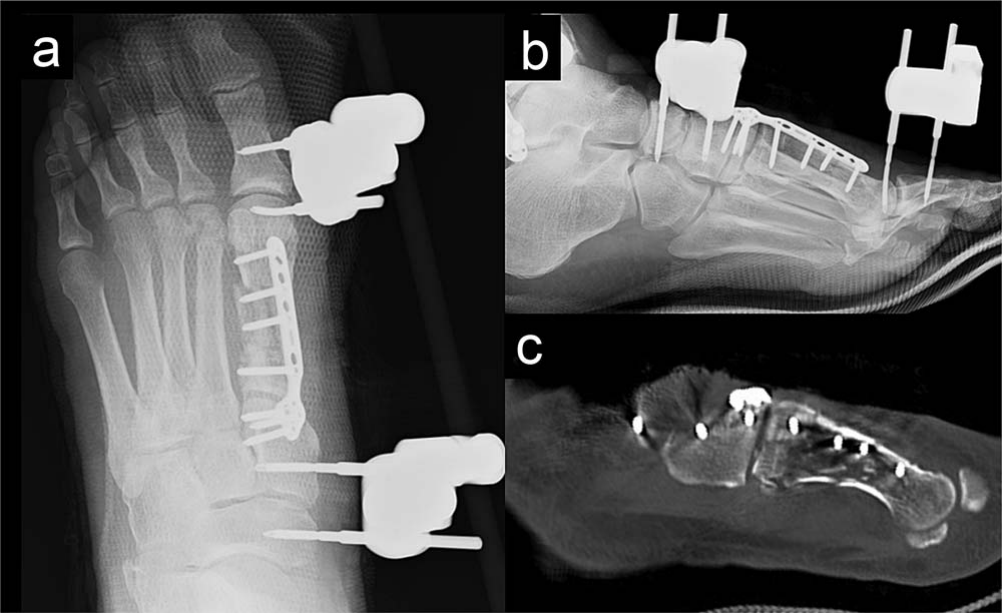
Radiography (a, b) and computed tomography (c) images immediately after the second surgery.
